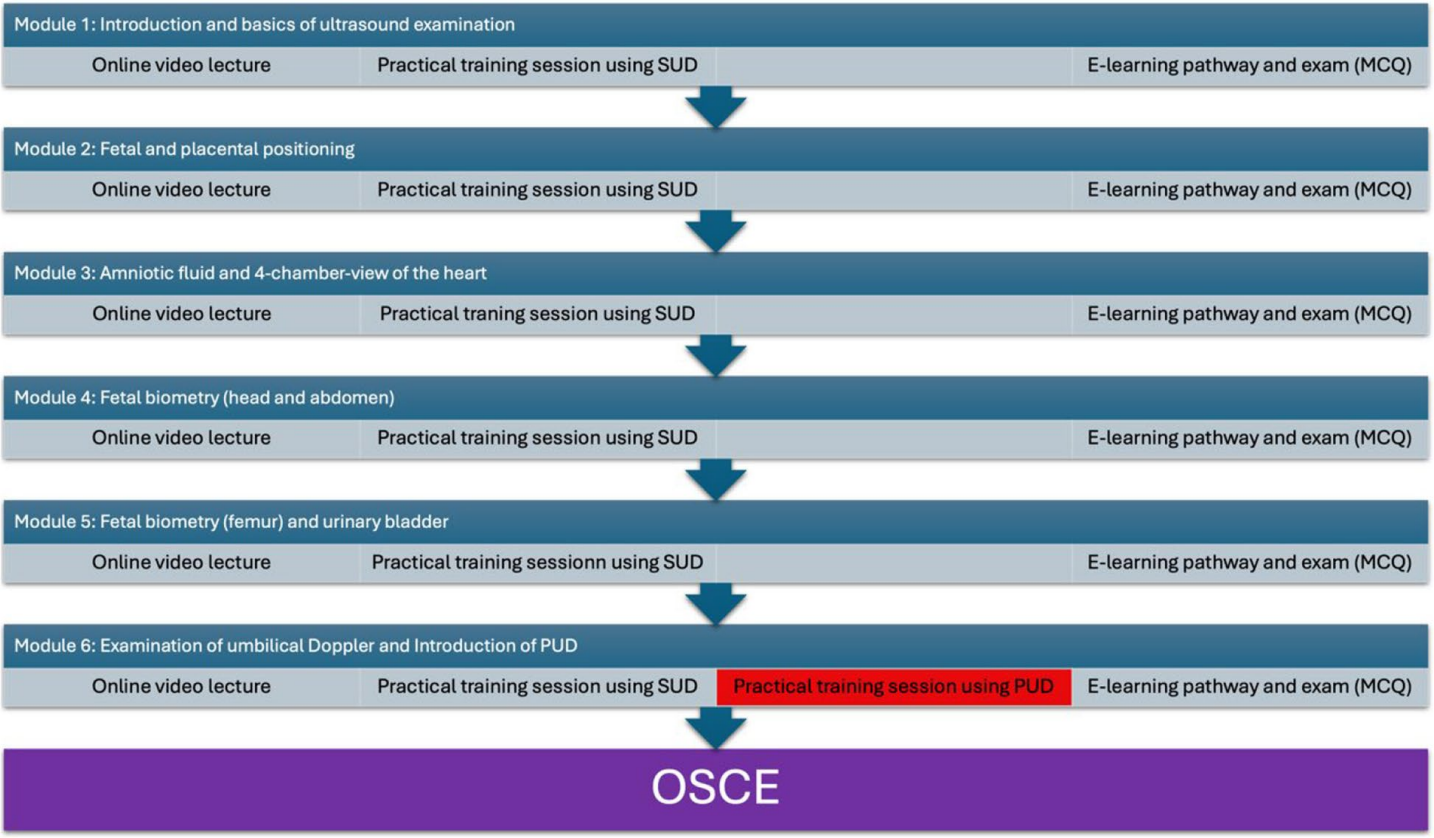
Overview of the training program of the students. MCQ: multiple choice question, OSCE: objective structured clinical examination, SUD: standard ultrasound device, PUD: portable ultrasound device
